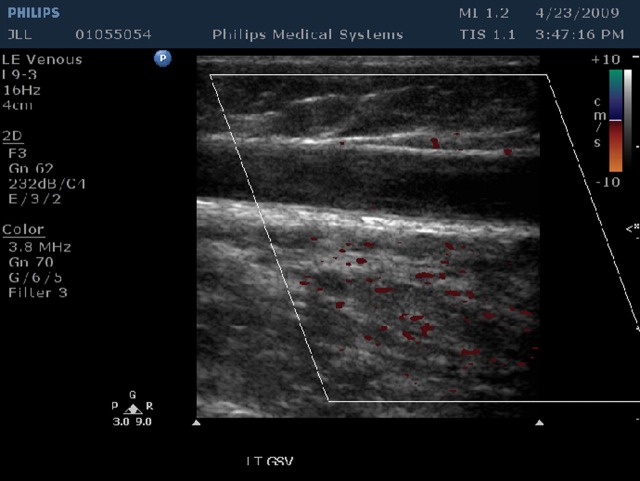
Ultrasound of closed GSV at one-week (no flow and no vein wall retraction). GSV, great saphenous vein
